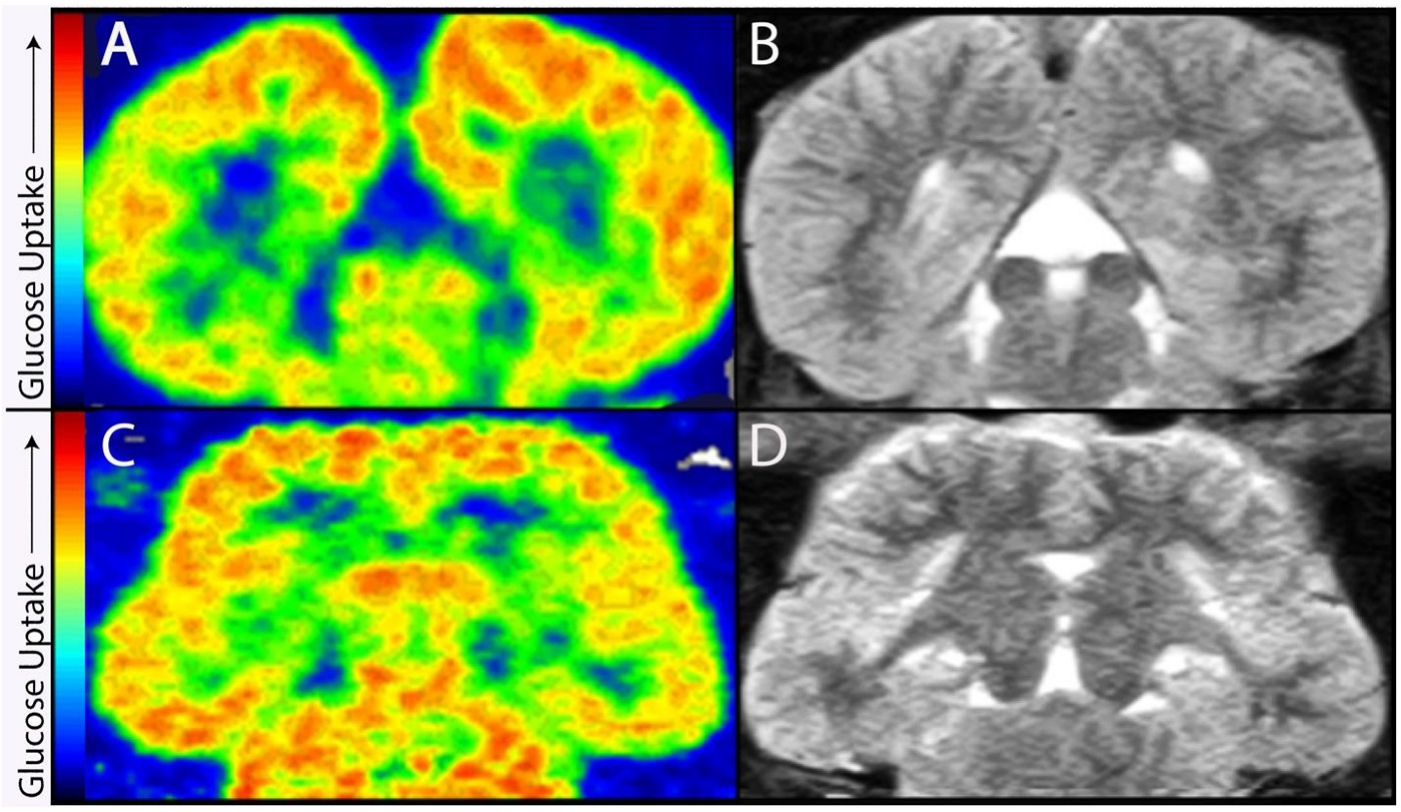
PET scans show that gray matter of the dolphin cerebrum and cerebellum have the highest metabolism in the brain. Frontal (A, B) and horizontal (C, D) views of a *Tursiops truncatus* brain after FDG PET (A, C) and MRI (B, D) scans. Images have been modified from previous publications with the authors’ permission [7,8]. The color map indicates the relative degree of glucose metabolism. The images demonstrate that high metabolic areas (i.e., areas of increased glucose consumption; red) are mainly concentrated in the gray matter of the cerebral cortex and cerebellum, with the exception of smaller sub-cortical nuclei (e.g., inferior colliculi; thalamic gray matter). The PET and MRI scans are from the same healthy dolphin that was trained to lie still in the scanner.
